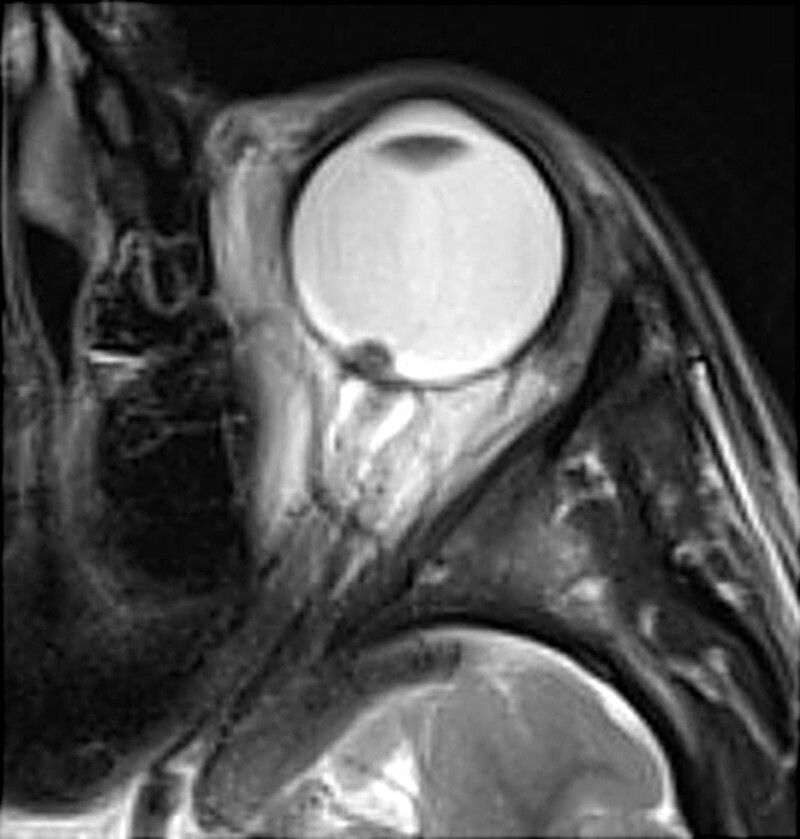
Magnetic resonance imaging of the head and orbits - 7-years follow-up.
